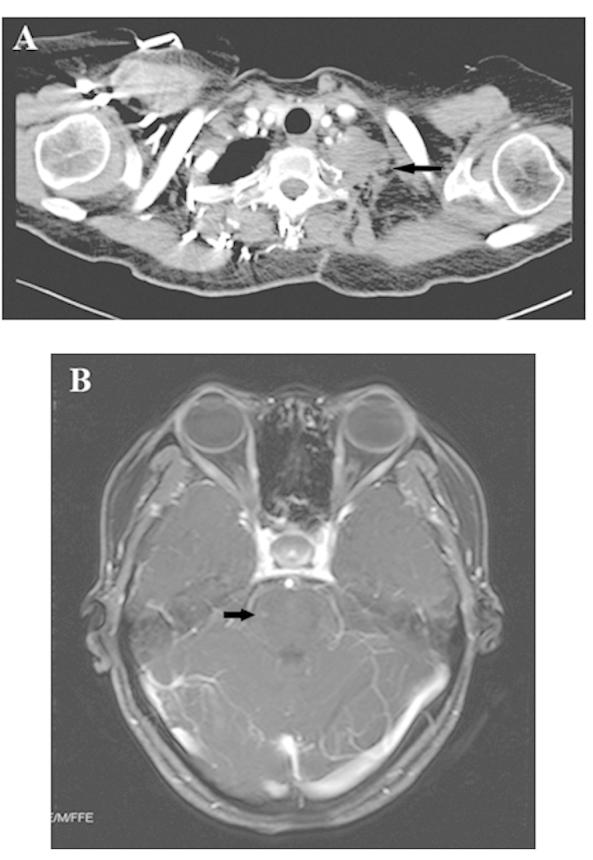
Computed tomography and brain magnetic resonance imaging of (A) the stable desmoid tumor in the lung (black arrow) and (B) the stable nodule (black arrow) in the right of the pons, respectively, at the 9-month follow-up in August 2013.
